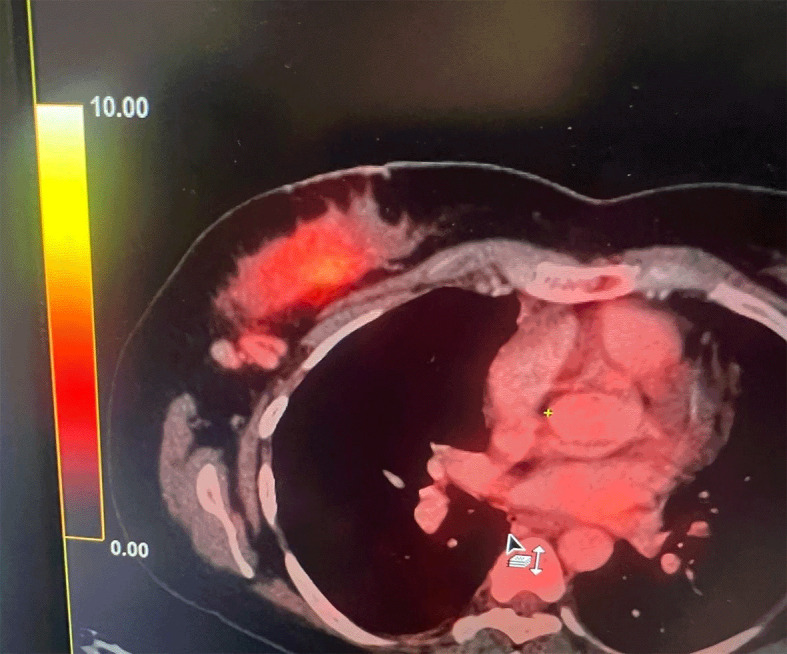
PET/CT image showing axillary node and primary tumor uptake.
